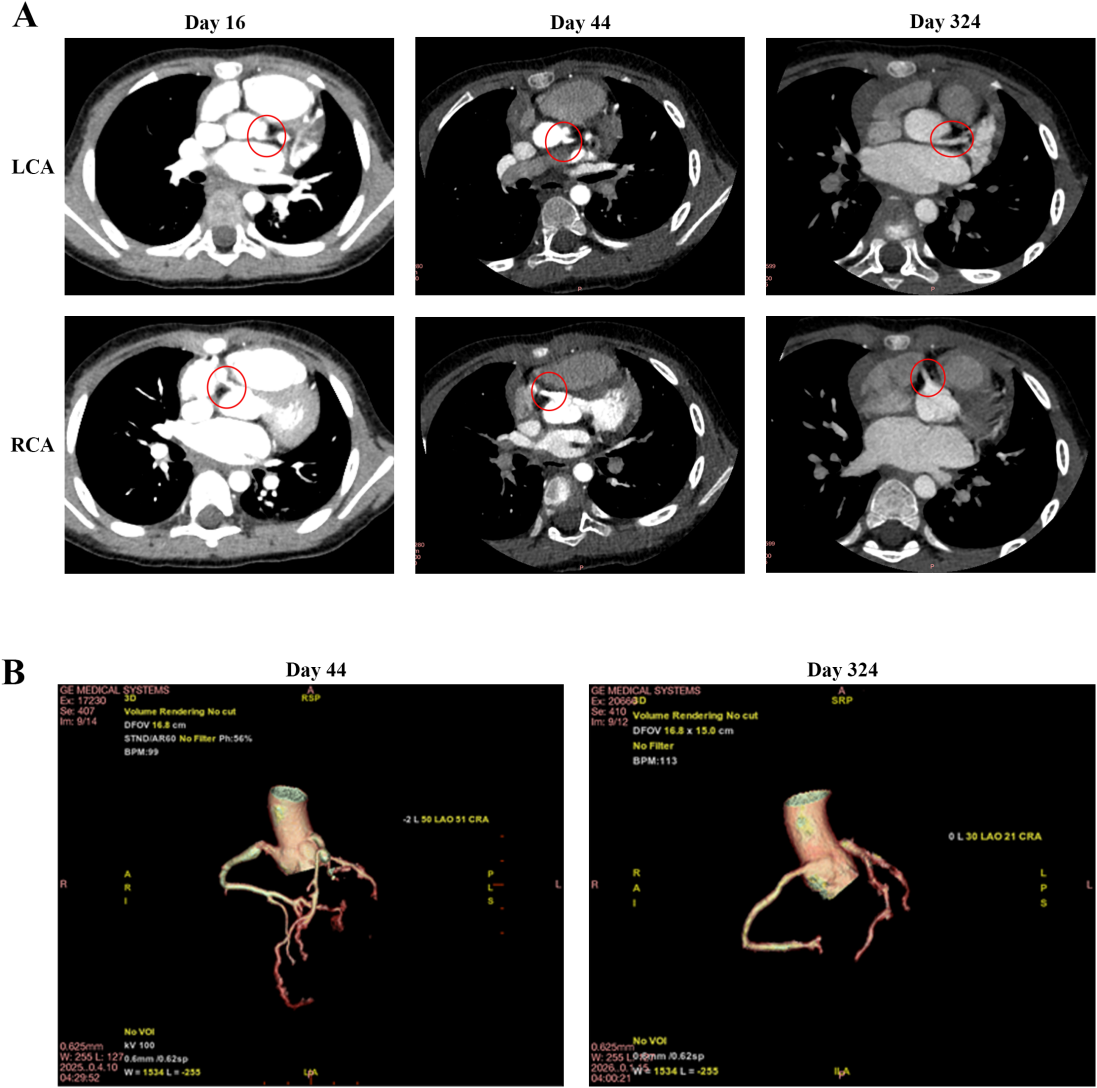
Radiological evaluation of giant CAA regression via coronary computed tomography (CT). (A) 2D axial imaging: Baseline plain CT (Day 16) and subsequent coronary computed tomography angiography (CCTA) (Day 44) demonstrate bilateral giant aneurysms (red circles). Follow-up imaging at Day 324 confirms complete morphological normalization with smooth vascular contours. (B) 3D coronary reconstruction: Spatial morphology of the coronary tree at Day 44 visualizes the extent of bilateral giant aneurysms. Follow-up reconstruction at Day 324 demonstrates anatomical restoration without residual stenosis or aneurysmal dilation.
